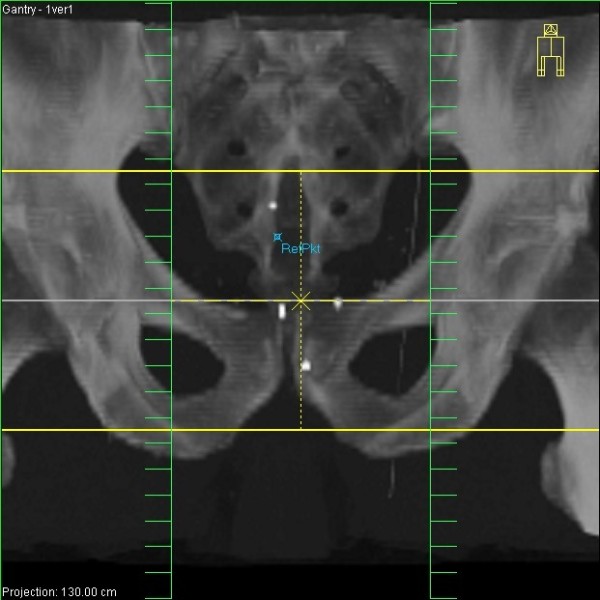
Visualization of three gold markers implanted into the prostate on a reconstructed CT image. The prostate itself is not visible, but the three intraprostatic markers can be used for daily image-guided radiotherapy (IGRT) with online adaptation of the beams to the current prostate position.
